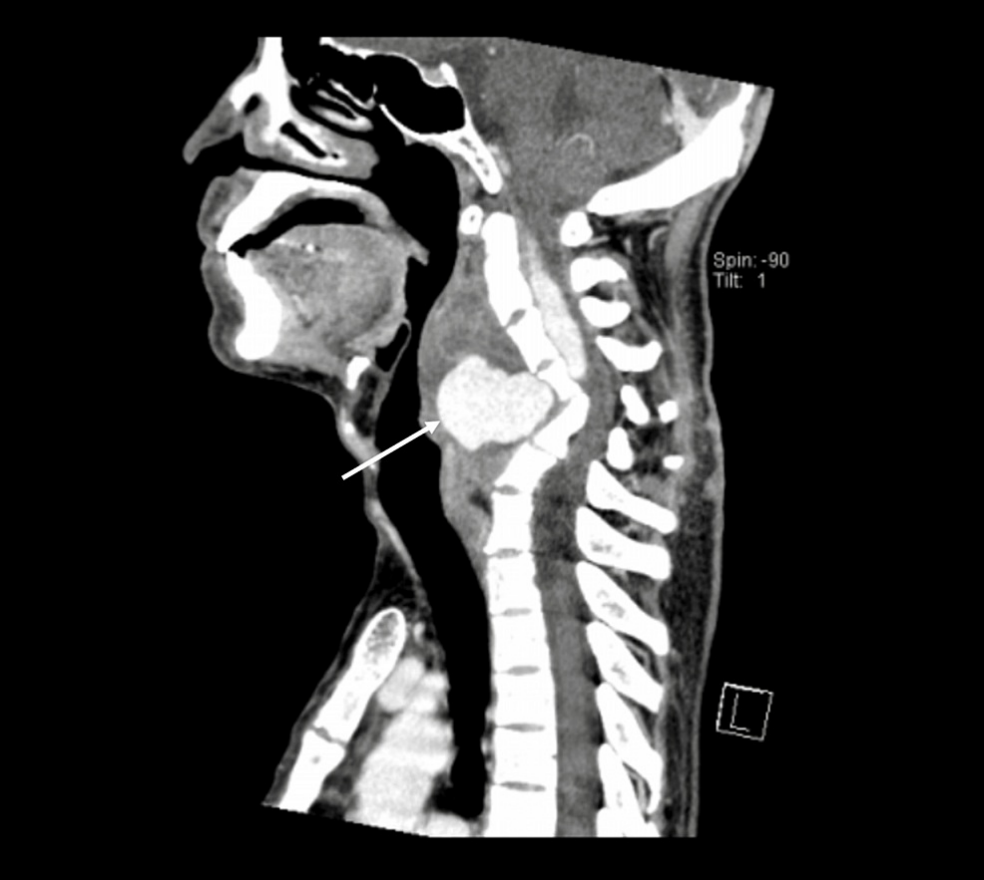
CTA vertebral artery. CTA vertebral artery in the sagittal plane showed the presence of a highly vascular lesion (thin arrow) suggestive of an aneurysm. CTA: computed tomography angiography.
